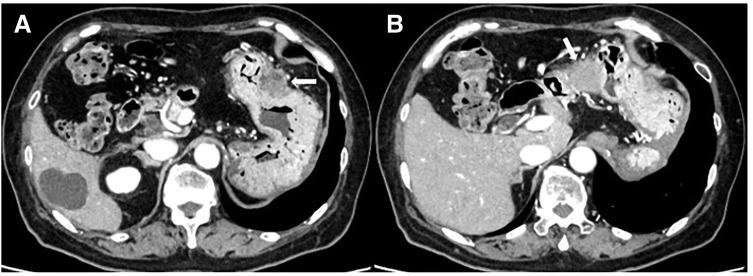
Three years and 6 months after initial pancreatectomy, CT revealed two low-density masses (A, B) in the wall of the stomach.
